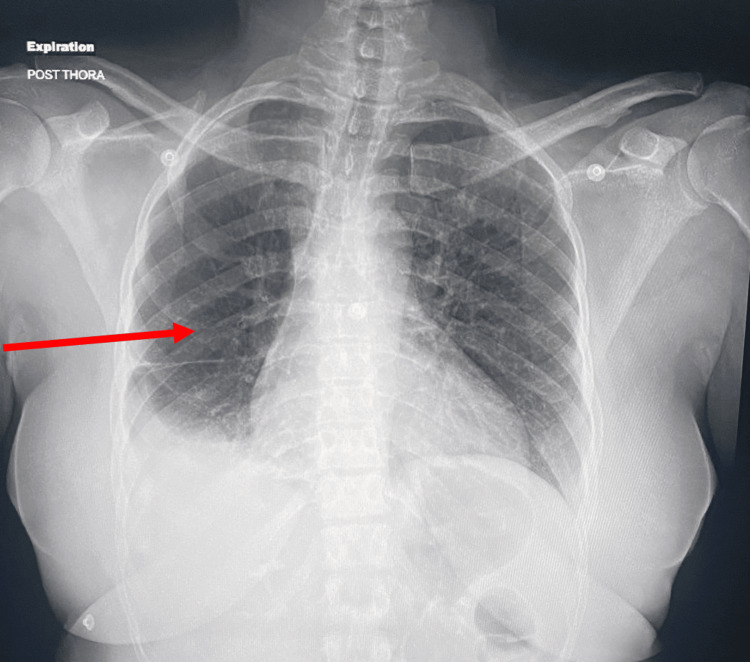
Chest Radiograph Showing a Significant Improvement in Right-Sided Pleural Effusion After Thoracentesis Red arrow highlighting the area of interest.
